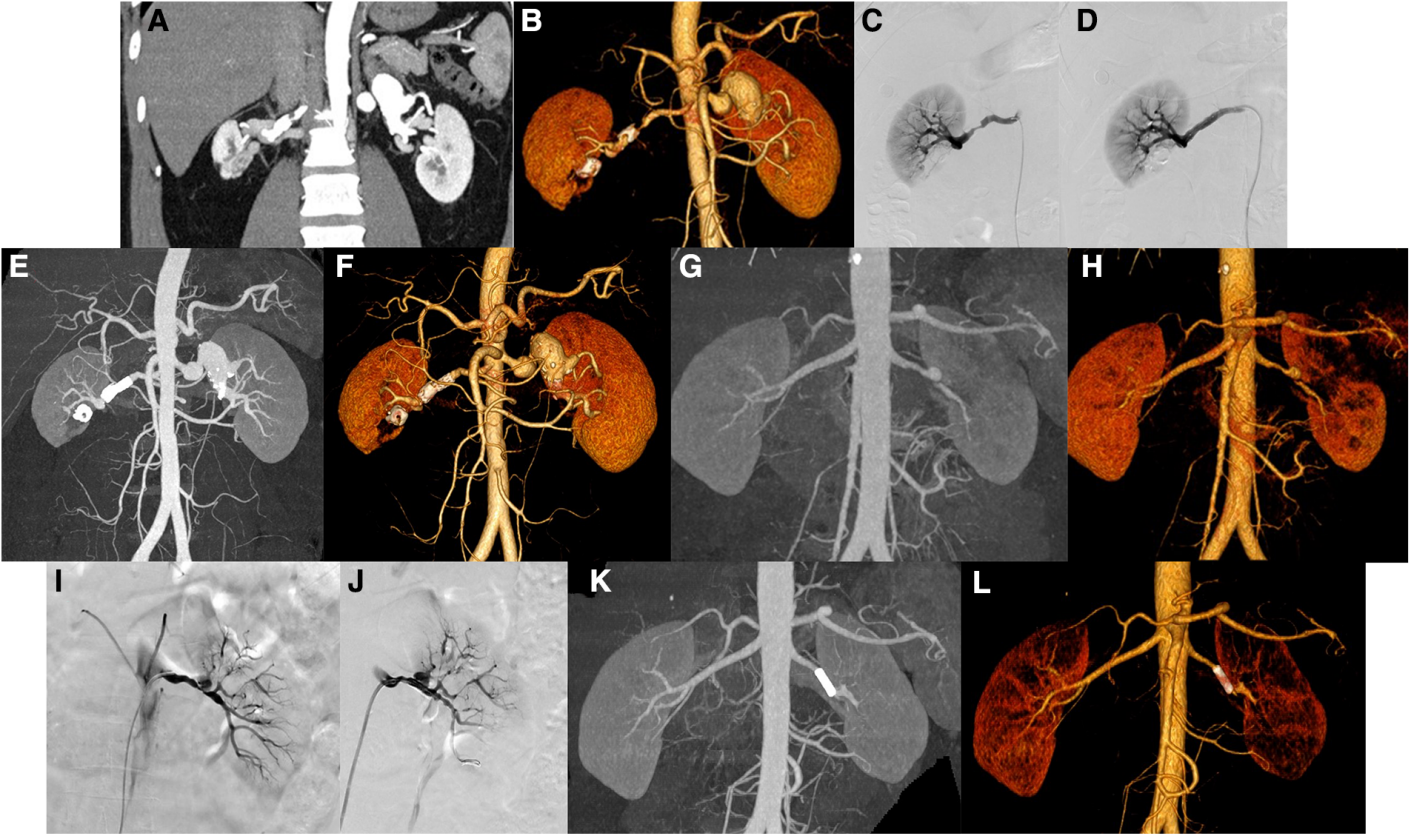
Patients no. 12 with renal aneurysms: (A,B) Computed tomography (CT) showed the main aneurysms of both renal arteries, with partial mural thrombosis and partial wall calcification, of which the main left renal artery is obvious (the widest part is approximately 2.5 cm in diameter). There are low-density foci in the lower right kidney near the renal sinus, with circular calcification at the edge, and adjacent right renal parenchyma with atrophy and ischemia. (C,D) The left and right renal arteries were performed, and frontal imaging (digital subtraction angiography) showed that the middle and distal parts of the left renal artery are enlarged (approximately 2.1 cm in diameter), involving intrarenal branches. There is approximately 60%–70% stenosis in the middle and distal part of the right renal artery, and the stenosis improved after stent implantation. (E,F) Three months later, CT showed that the stent lumen of the right renal artery is unobstructed. Patients no. 10 with renal aneurysm: (G,H) CT showed local dissection or formation of an aneurysm in the main trunk of the left renal artery and multiple ischemic abnormal enhancement areas in the left kidney. (I,J) Left renal artery angiography showed that the distal part of the left renal artery is approximately 80%–90% stenosis and a mung bean-sized aneurysm was observed. There is also 70%–90% stenosis in multiple branches of the left kidney. After stent implantation, the stenosis of the left renal artery is improved and covered the neck of the aneurysm. (K,L) CT scan 1 year after the operation showed that the left renal artery stent is in place, and the lumen is unobstructed.
